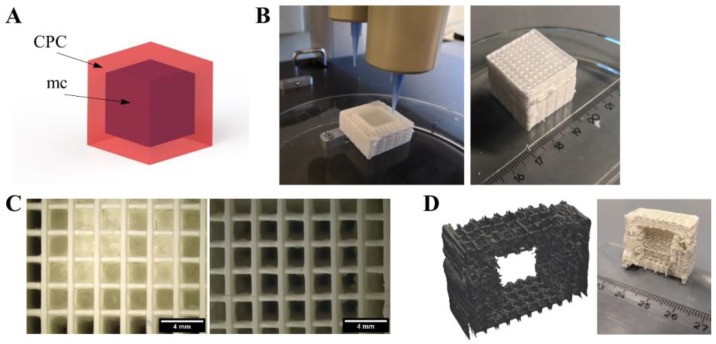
Generation of an inner cavity inside a CPC scaffold. (A) Principle of the CPC (red) structure with an inner cavity, filled with support material (mc, purple). (B) Fabrication and post-plotting appearance of the cubic structure with 410 μm needles. (C) After plotting (left), pores in *z*-direction of the CPC part are not interconnected but closed by mc at the interface of both materials. After methylcellulose vanished (right), the pores were clearly open and interconnected (scale bars 4 mm). (D) Virtual cut of micro computed tomography of the cube and photograph of the cut cube after mc vanished, revealing the cubical-shaped cavity inside the macroporous CPC structure. More images are provided in Supplementary Figure S1.
